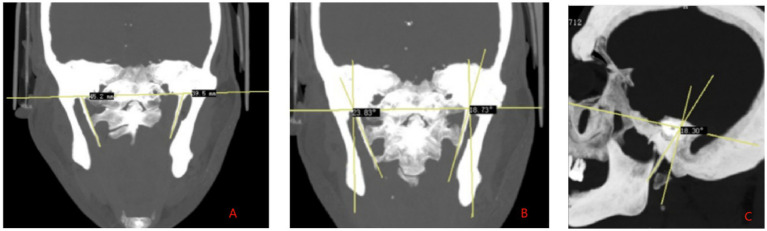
High-resolution CT three-dimensional reconstruction maximum density projection. (A) SP length measurement. (B) SP measurement of medial declination angle. (C) SP measurement of anterior inclination angle.
